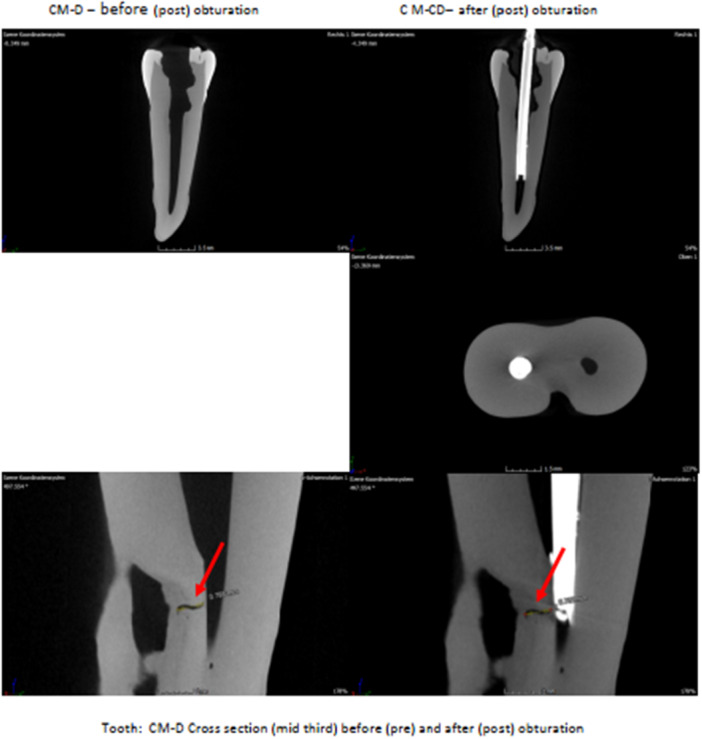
CT Images of teeth obturated. N.B.: Red arrows indicate tooth fractures before and after obturation. No microcrack propagation was observed.
